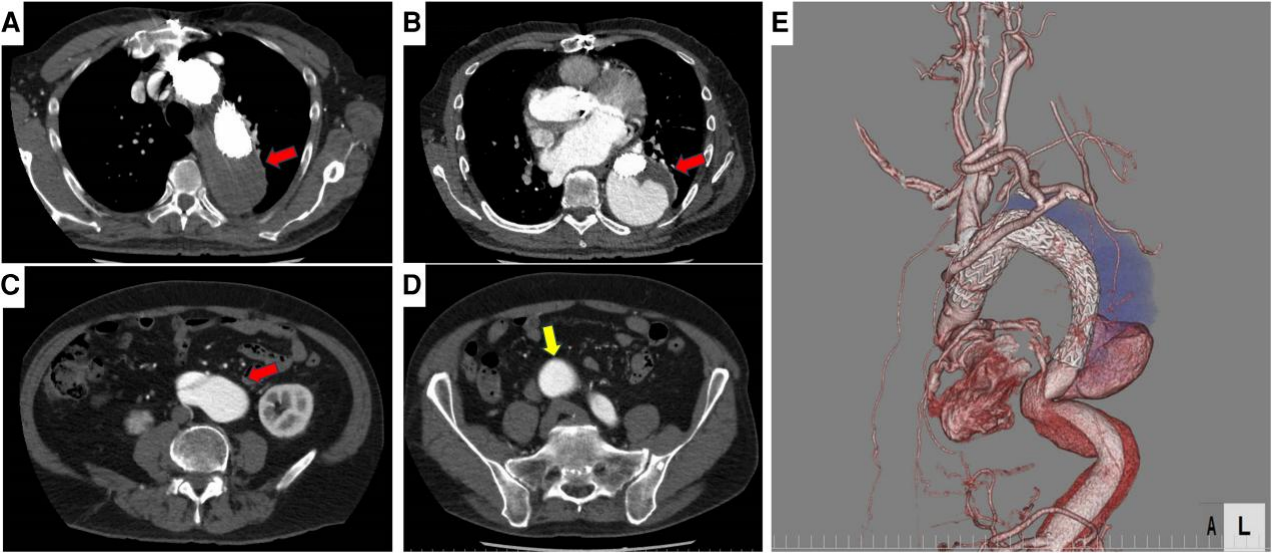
CT scan findings of dissecting aortic aneurysm. The contrast CT scan shows a thoracoabdominal dissecting aortic aneurysm with a partial false lumen opening (71 mm of maximum short diameter), and exhibiting a saccular shape (red arrow in panels *A–C*). There are residual abdominal aorta dissection and a right common iliac aneurysm (yellow arrow in panel *D*). The aortic arch is repaired with a stent graft. (*A*) Cross-section of the aortic arch, (*B*) cross-section of the thoracic descending aorta, (*C*) cross-section of the infrarenal aorta, (*D*) cross-section of the bifurcation of the common iliac artery, (*E*) 3D-CT angiography at chest level.
